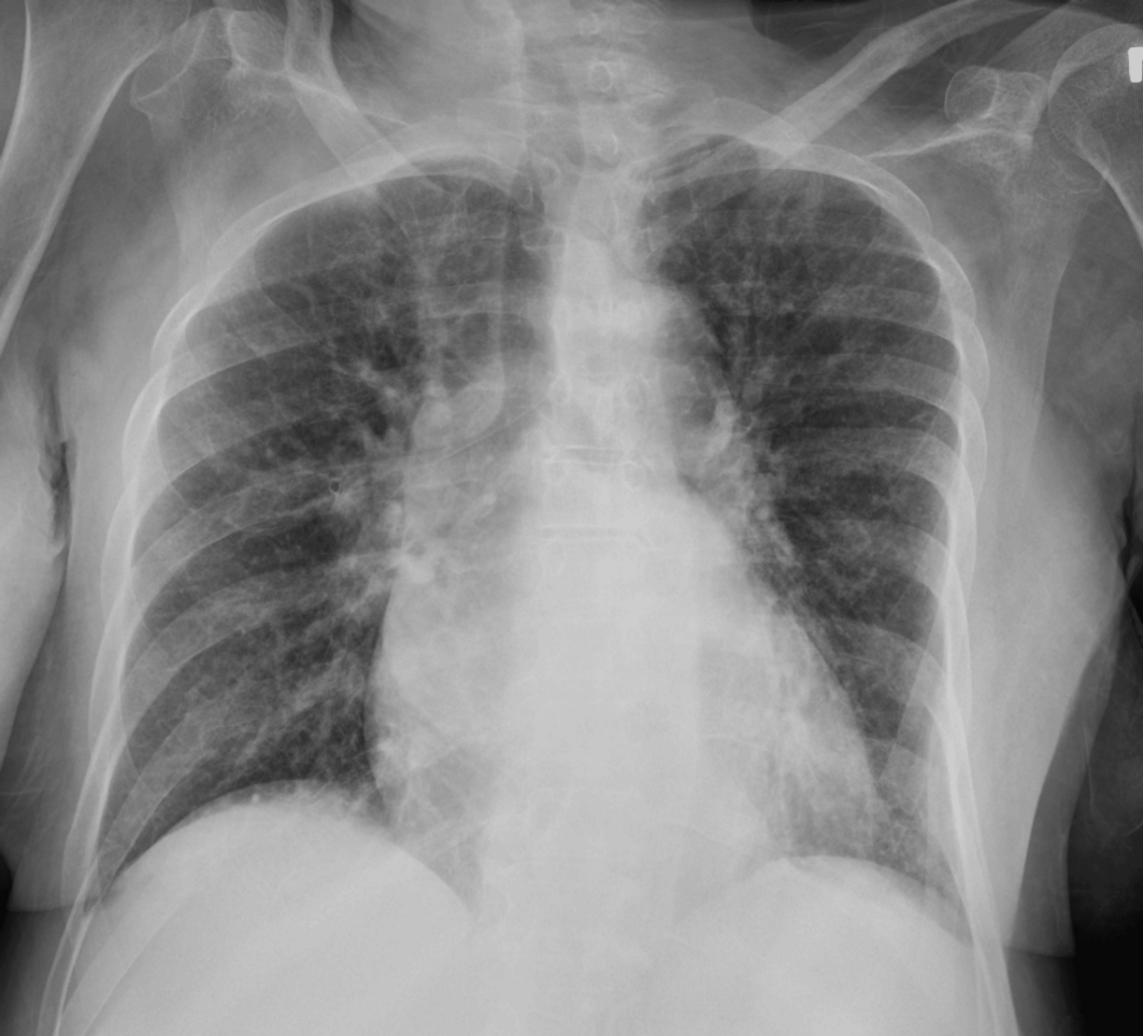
X-ray (antero-posterior view) showing no pulmonary pathology
